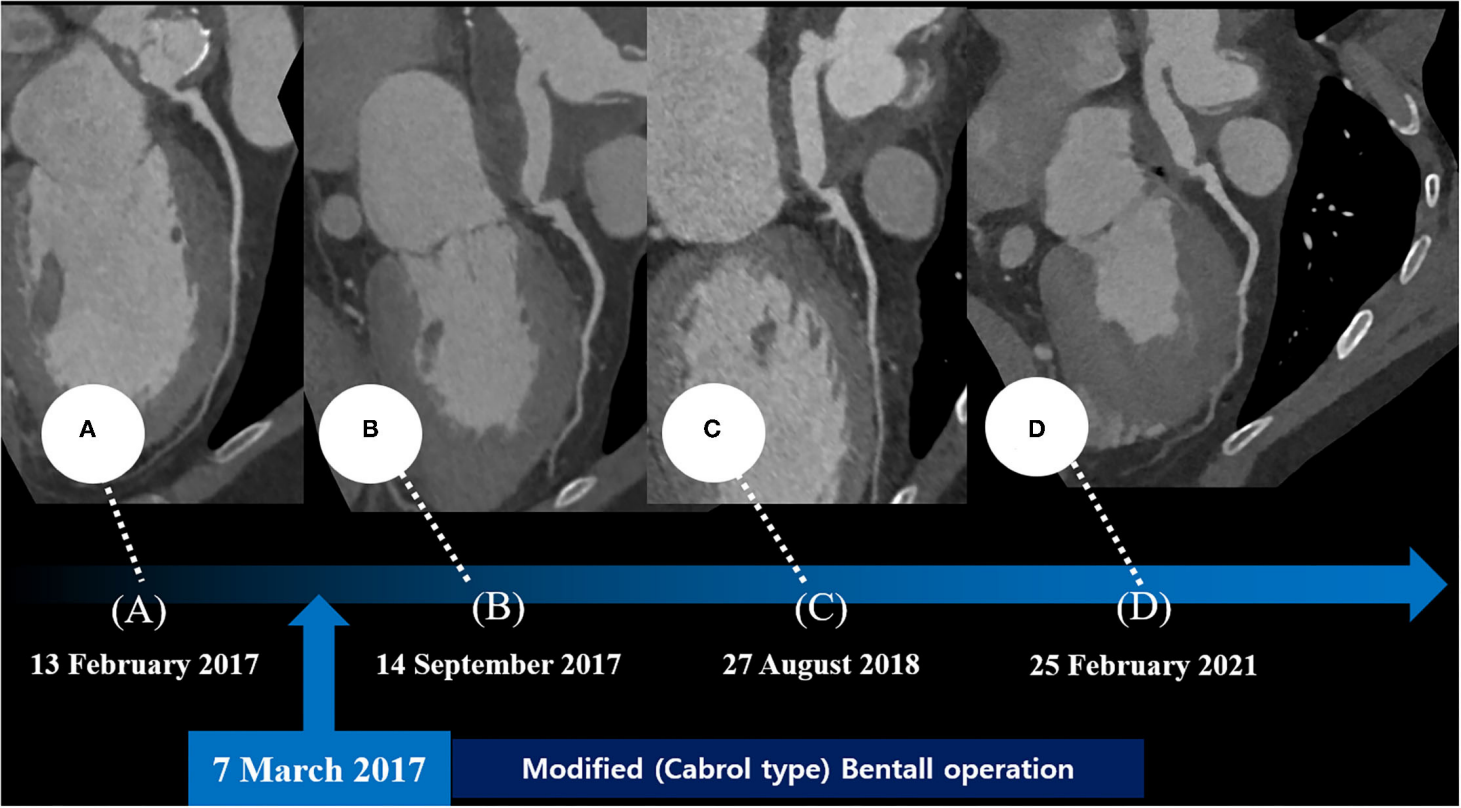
Series of coronary computed tomography angiographies at the preoperative period (A) and the postoperative period (B–D).
